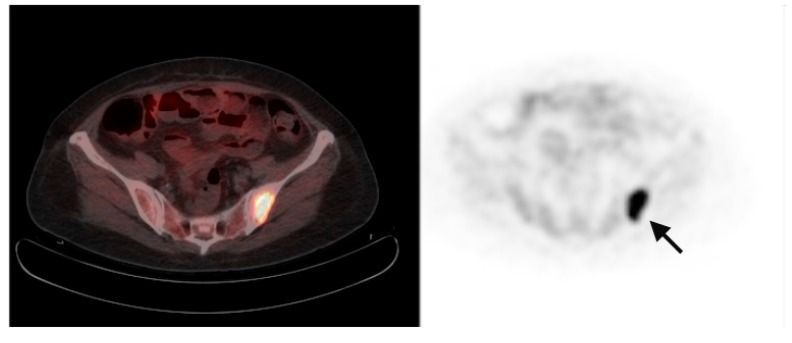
Metastatic HCC. Corresponding PET/CT (left) and PET (right) images show abnormal increased FCh uptake in the left posterior ilium (*arrow*) consistent with the diagnosis of skeletal metastasis.
